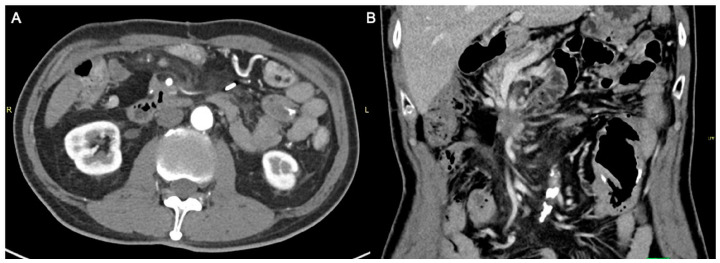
Axial (A) and coronal (B) computed tomography images demonstrating the remnant pancreatic tumor, now smaller in size and extent, measuring approximately 2.3 cm.
